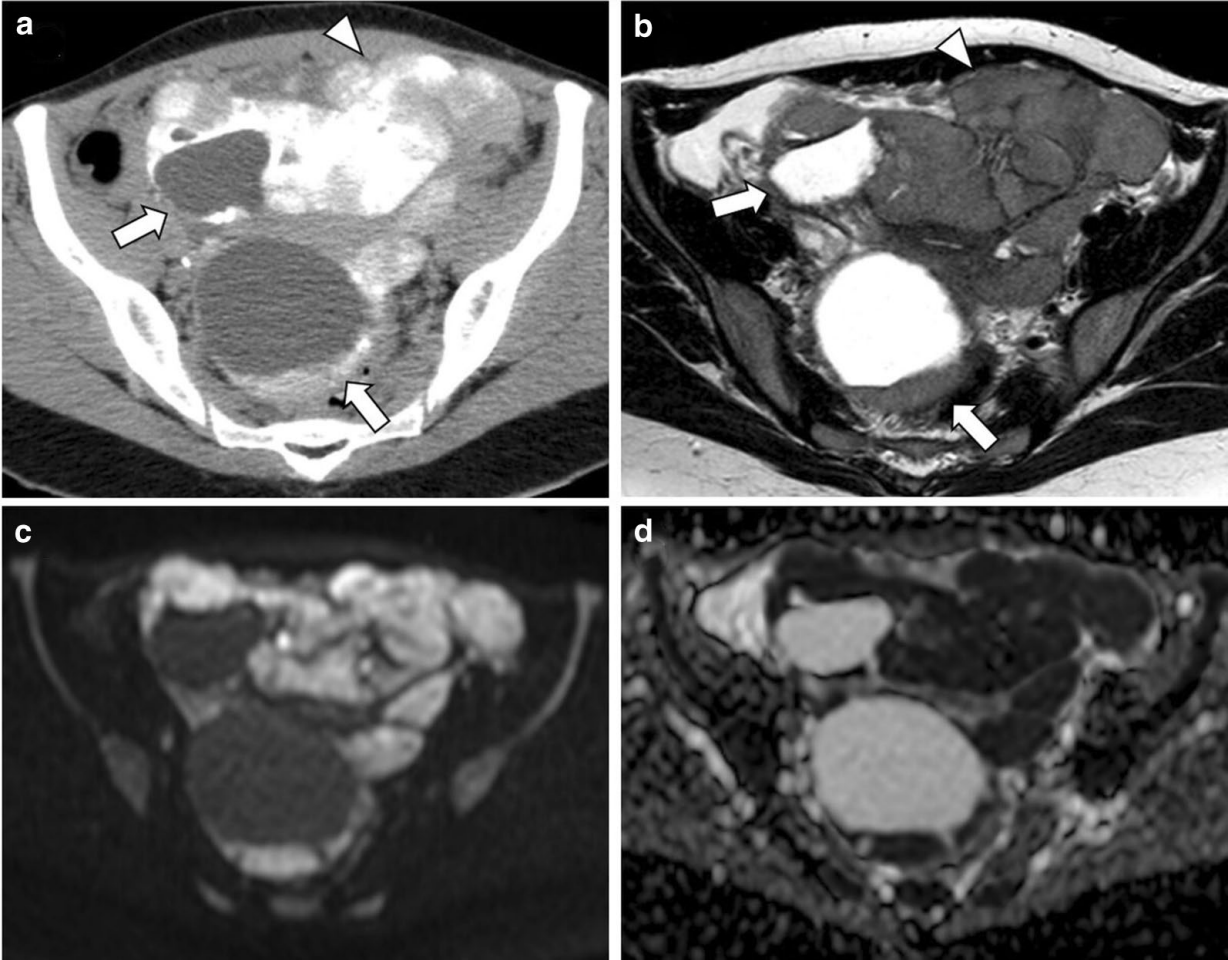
Bilateral ovary LGSC with multiple peritoneal metastases in a 22-year-old female. Axial unenhanced CT image (a) and axial T2-weighted MR image (b) demonstrate a complex bilateral mixed ovarian tumour, with solid parietal components (arrows) and multiple pelvic solid metastases with exuberant calcified psammoma bodies (arrowheads). Axial *b*-1000 s/mm^2^ diffusion-weighted image (c) and ADC map (d) show diffusion restriction in parietal nodules and peritoneal metastases
